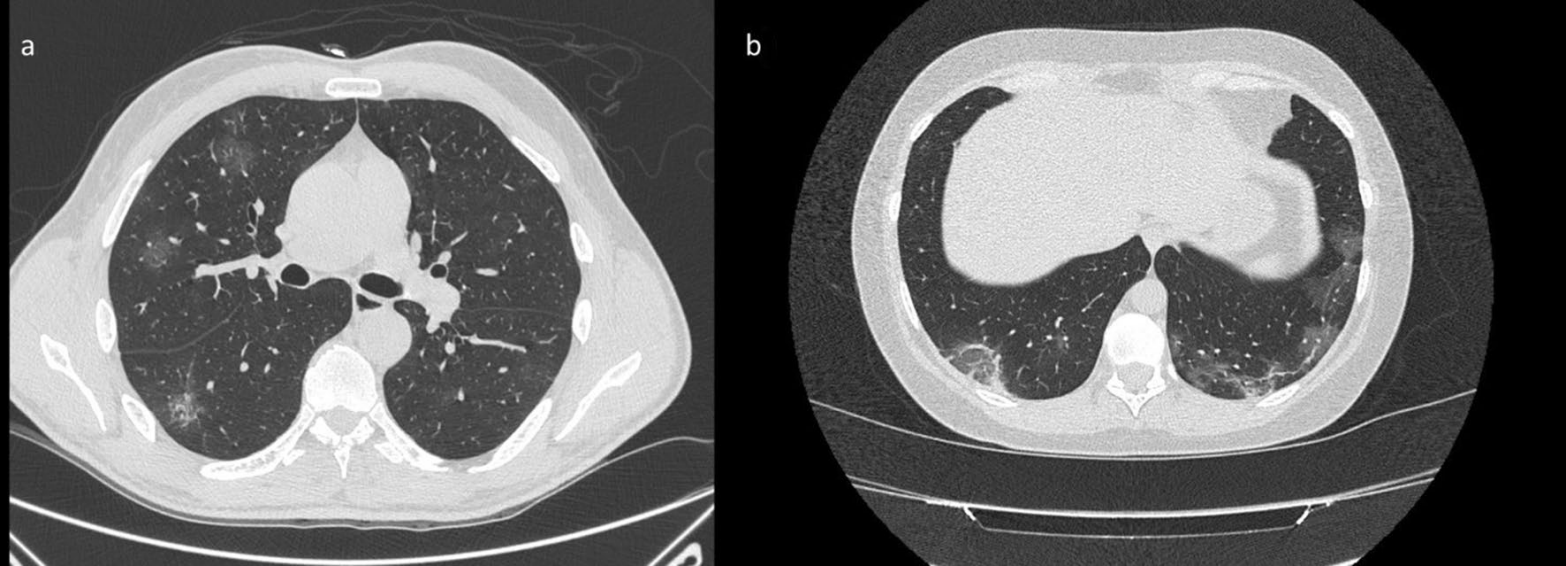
(a) CT scan shows multiple areas of GGO in the right upper and lower lobes with typical rounded morphology; (b) CT scan thought lower lobes shows bilateral involvement of posterior subpleural region of both lungs with areas of GGO and mild reticular pattern. Note the presence of “reversed halo sign” in the posterior segment of the right lower lobe.
